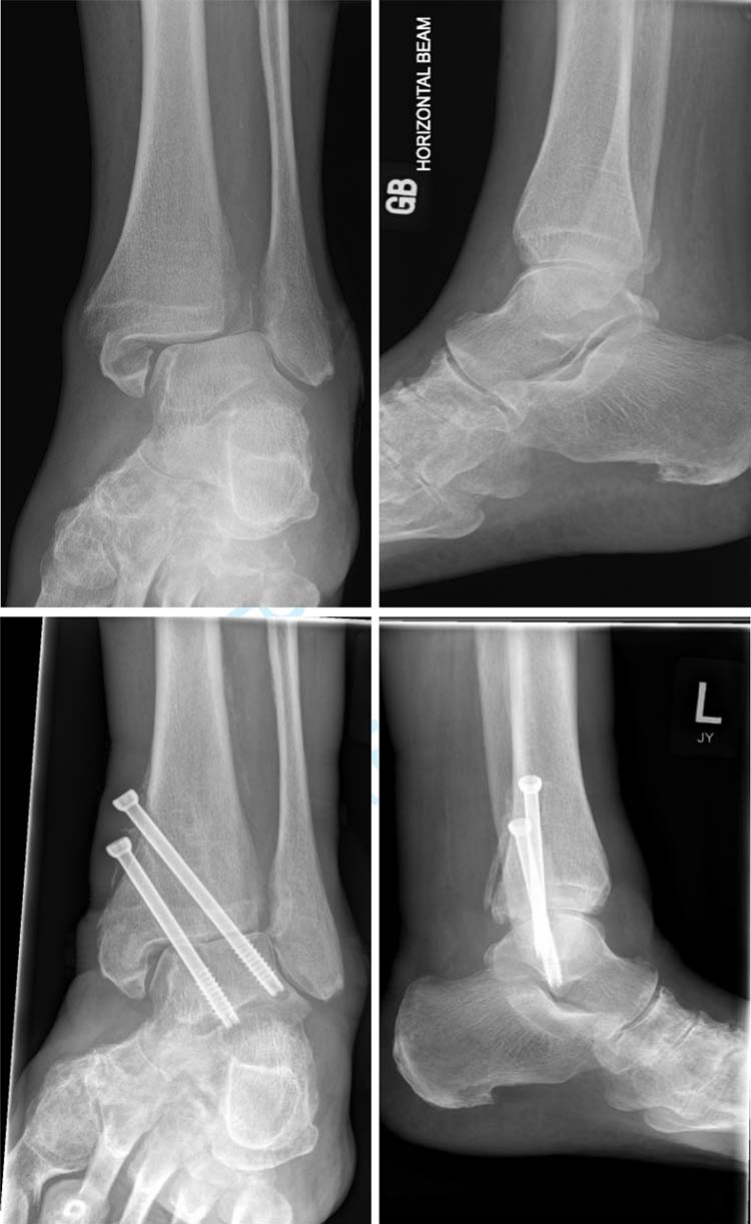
Top—Bimalleolar ankle fracture with talar shift. Bottom—Radiograph at 5 months showing small amount of talar shift.
